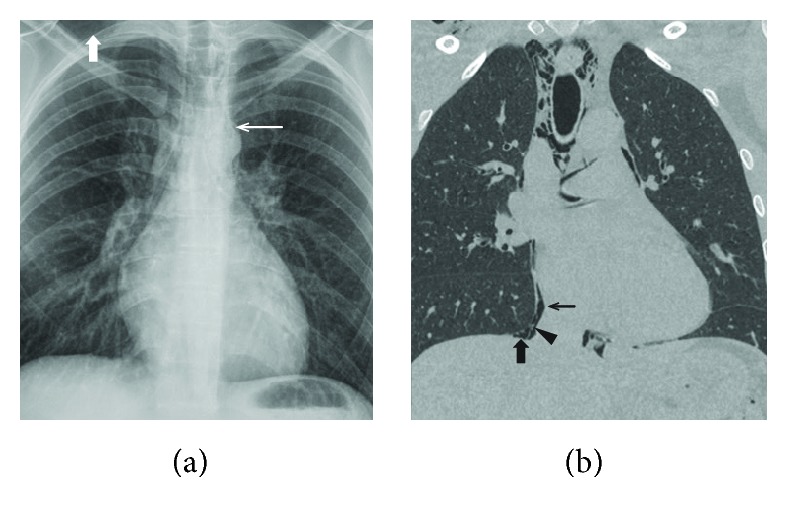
Chest X-ray (a) and chest CT (b). On both pictures, subcutaneous emphysema is apparent at the right side of the neck (thick white arrow) as well as air tracking in the centre of the mediastinum indicative for mediastinal emphysema or pneumomediastinum (thin white arrow). Chest CT reveals a pneumopericardium (thin black arrow) and a small pneumothorax (thick black arrow). The pericardium can be seen between the pneumopericardium and pneumothorax (black triangle).
